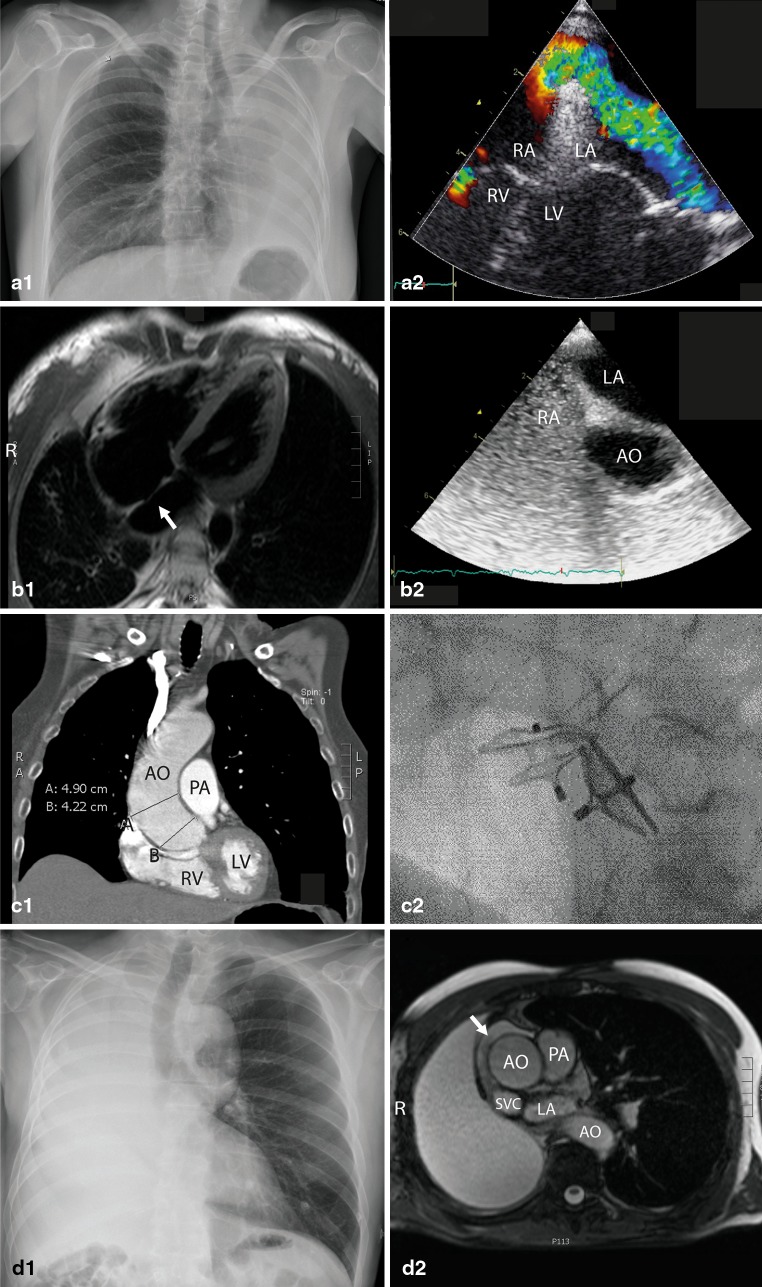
Different imaging modalities of case 1(a), 2 (b), 3(c) and 4 (d), respectively, for the purpose of diagnosing POS by PFO. a1 Chest X-ray showing the heart position against the left thoracic wall. a2 Four chamber view of a Doppler TEE with a right-to-left shunt by PFO. b1 CMR showing malposition of the right thoracic wall resulting in a heart shift to the right and presence of PFO (*arrow*). b2 Short axis basal view of a Bubble contrast TEE showing no resting right-to-left shunting over the PFO. c1 CT angiography showing the dilated ascending aorta (*A*) and aorta root (*B*). c2 Periprocedural angiographic image of both Amplatzer devices. d1 Chest X-ray showing a tracheal shift to the left after pneumonectomy. d2 CMR shows a right hemithorax filled with pleural effusion and a compressed right atrium (*arrow*). *RA* right atrium, *LA* left atrium, *RV* right ventricle, *LV* left ventricle, *R* right, *AO* aorta, *PA* pulmonary artery, *SVC* superior vena cava
